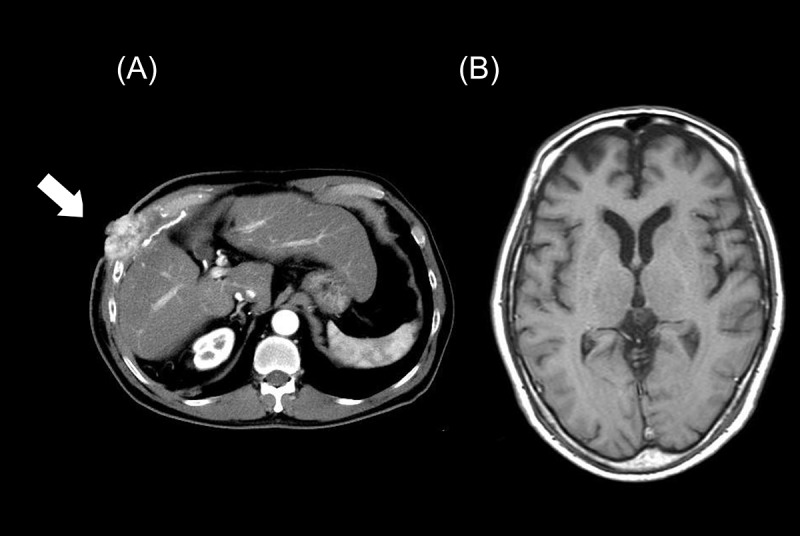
Patient imaging findings. (A) Abdominal CT findings. The white arrow indicates chest wall metastasis. (B) Head MRI findings. No brain metastases were observed.
